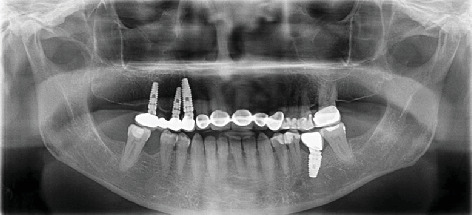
Panoramic radiograph after implant loading.
